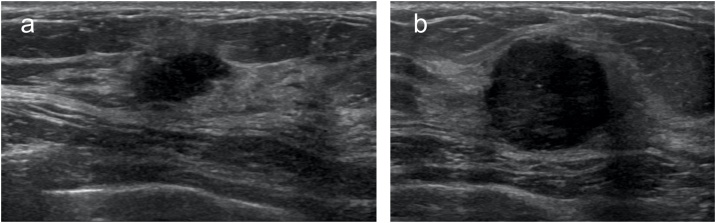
Breast ultrasound images at the first visit. a) The tumor on the right is a hypoechoic mass with unclear boundaries, irregular margins, and non-uniform internal echo. b) The tumor on the left is a well-defined, hypoechoic mass with irregular margins and relatively uniform internal echo.
